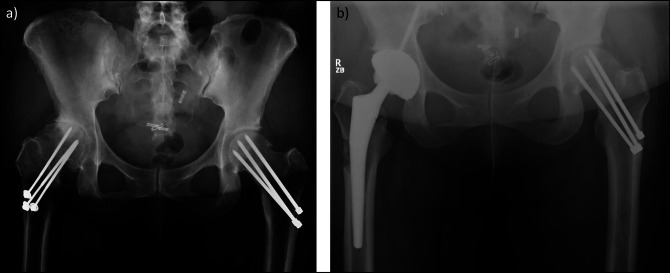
A, Adult AP pelvis radiograph showing retained Knowles pins in bilateral hips for the treatment of slipped capital femoral epiphysis 40 years earlier. B, AP pelvis radiograph after total hip arthroplasty requiring a significant amount of bone to be removed from the lateral cortex of the femur to allow for the removal of the pins.
